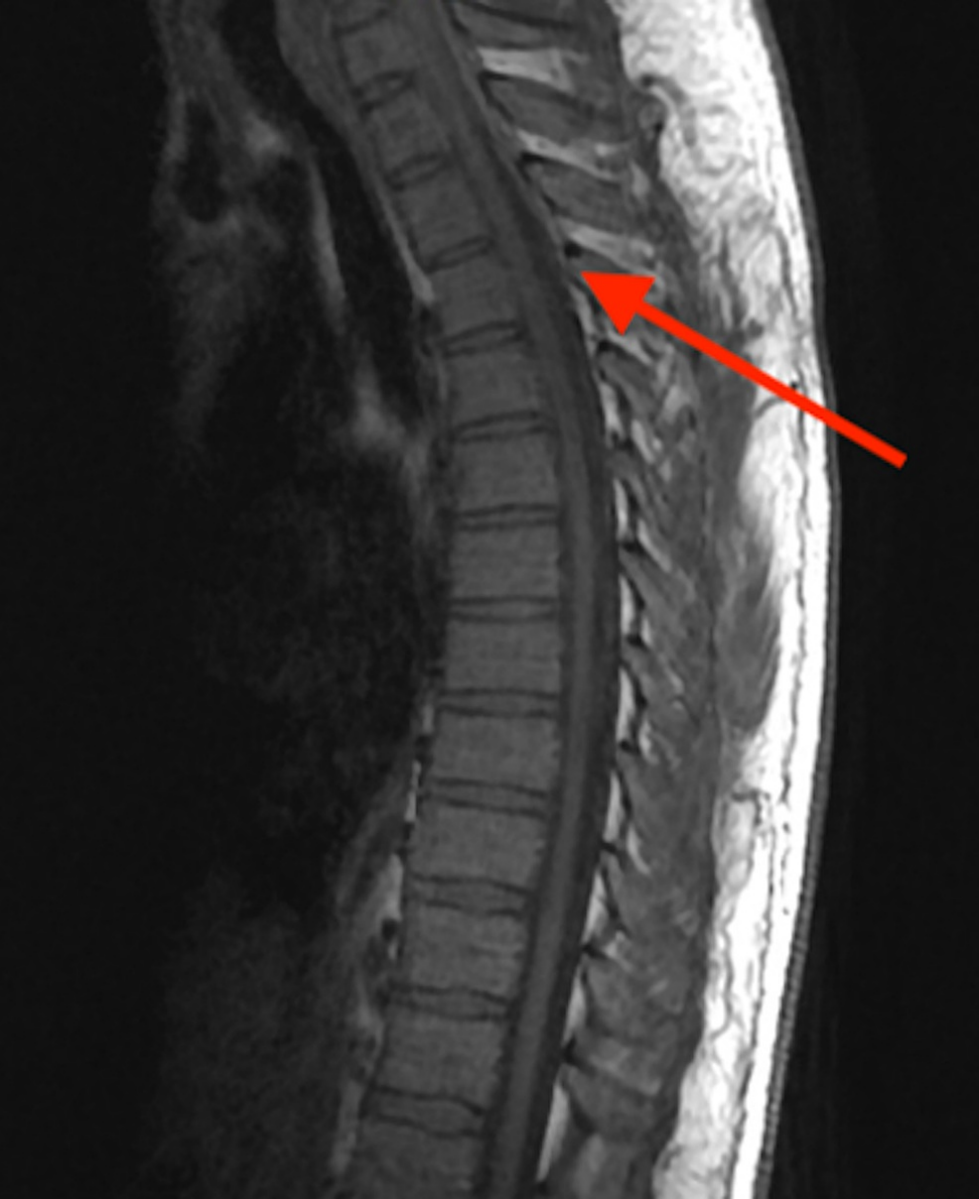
Sagittal T2 enhanced thoracic MRI showing severe spinal canal stenosis at T1 and moderate spinal canal stenosis at T2
